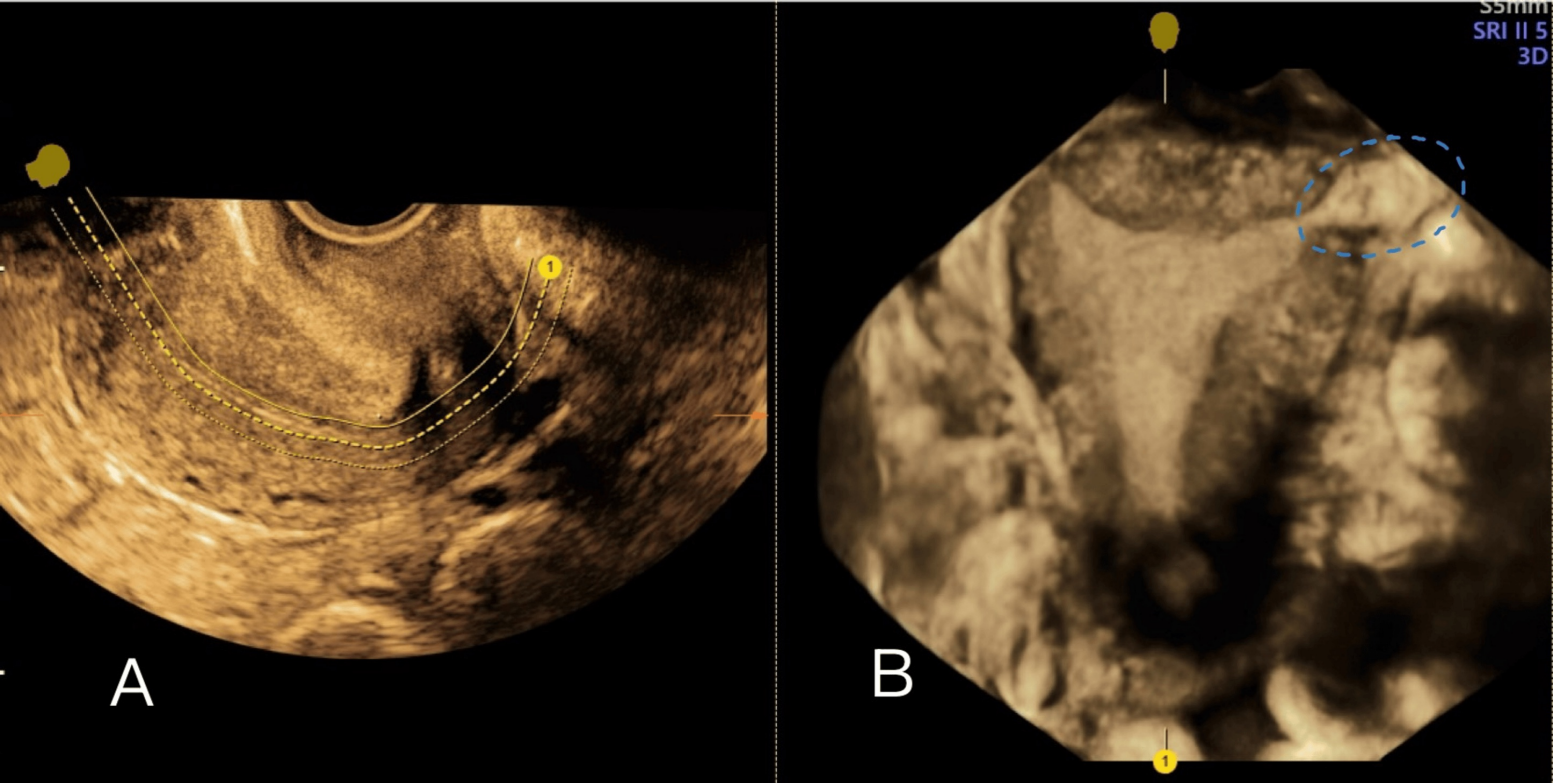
3D ultrasound surface rendering: A) empty uterine cavity and B) gestational sac in the left uterine cornu
